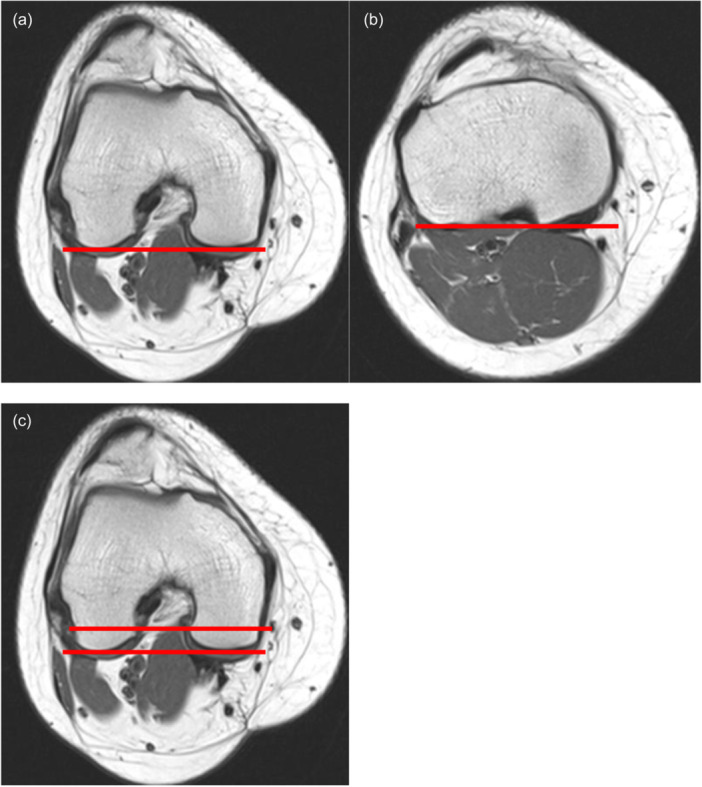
The figure shows the measurement of the TFR angle on MRI, right knee. (a) The posterior femoral condylar line is drawn at the level of the widest anterior–posterior diameter of the condyles, in the closest slice where both posterior condylar cartilages could be appreciated. (b) The posterior tibial condylar line is drawn at the first slice below the menisci, at the level of the posterior cruciate ligament insertion. (c) The two images were superimposed. The TFR angle was calculated as the angle between the two lines (in this example 0°). MRI, magnetic resonance imaging; TFR, tibiofemoral rotation.
